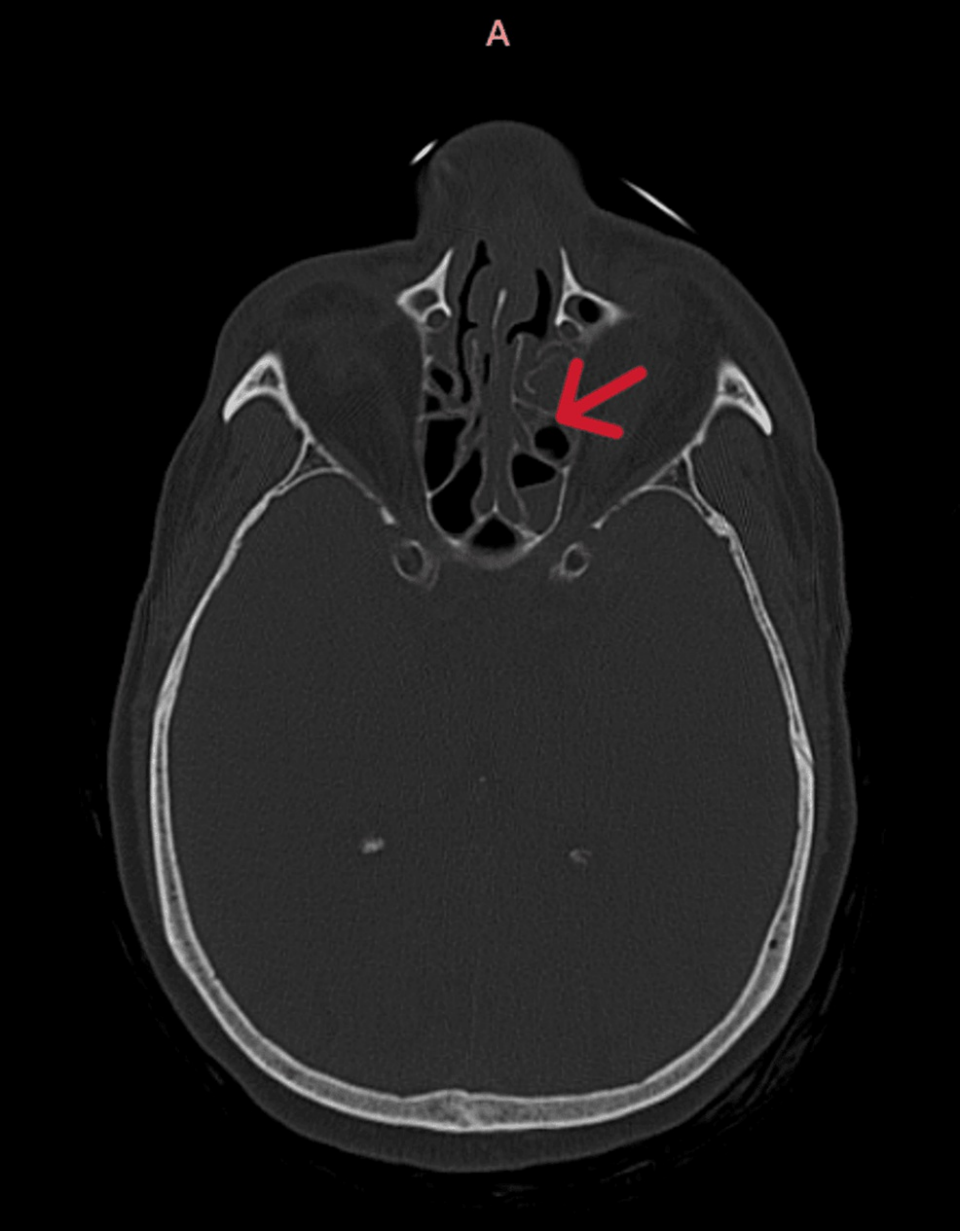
Non-contrast CT (NCCT) brain bone window showing ethmoidal air cells (red arrow) Mucosal thickening of bilateral ethmoidal air cells is seen in this scan.
